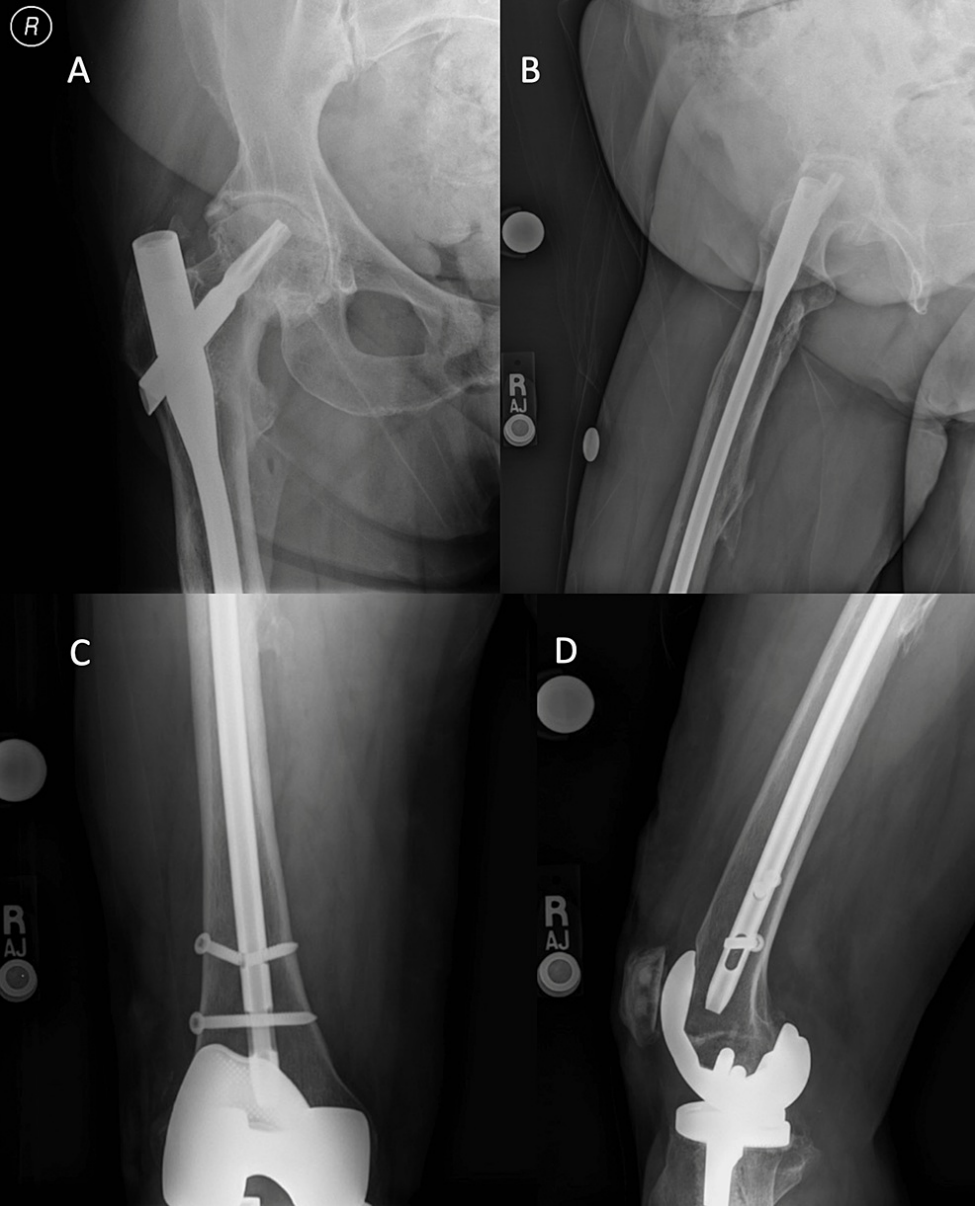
Preoperative radiographs Anteroposterior (A,C) and lateral (B,D) right femur radiographs demonstrating a healed subtrochanteric femur fracture with autodynamization of the distal interlocking screws and advanced right hip degenerative joint disease.
